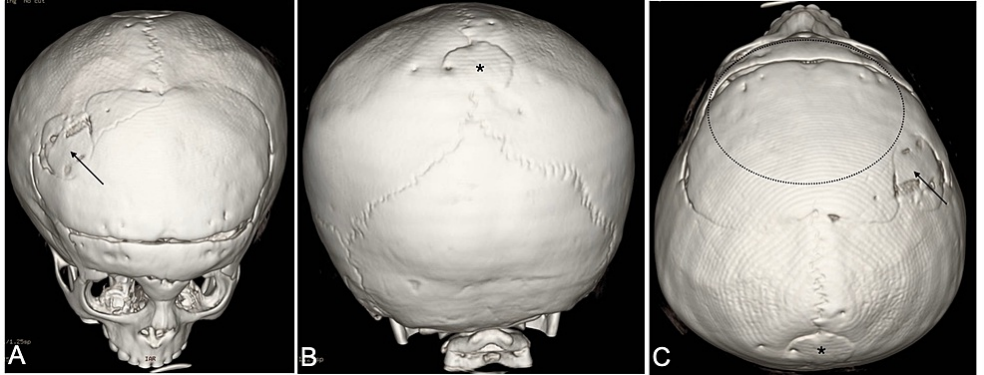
Computerized tomography of skull one year later of reconstructive surgery and treatment of trigonocephaly and cranium bifidum occultum This 3D reconstruction of a CT scan 12 months after surgery reveals the proper ossification of the autologous bone grafts in the frontal bone (thin black arrow) and cranium bifidum (*), as well as the reshaping of the skull, which acquired a round shape (dot ellipse) with the resolution of trigonocephaly.
